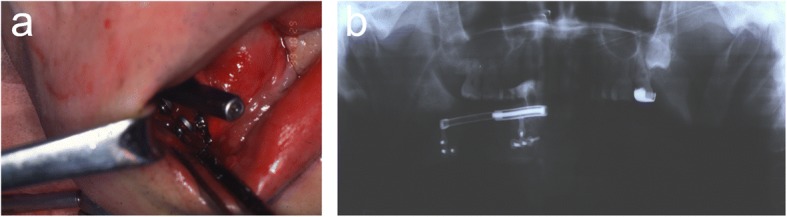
a Horizontal distraction osteogenesis of the remaining mandible was performed to reduce the bone defect. b Orthopantomogram after horizontal mandibular distraction
